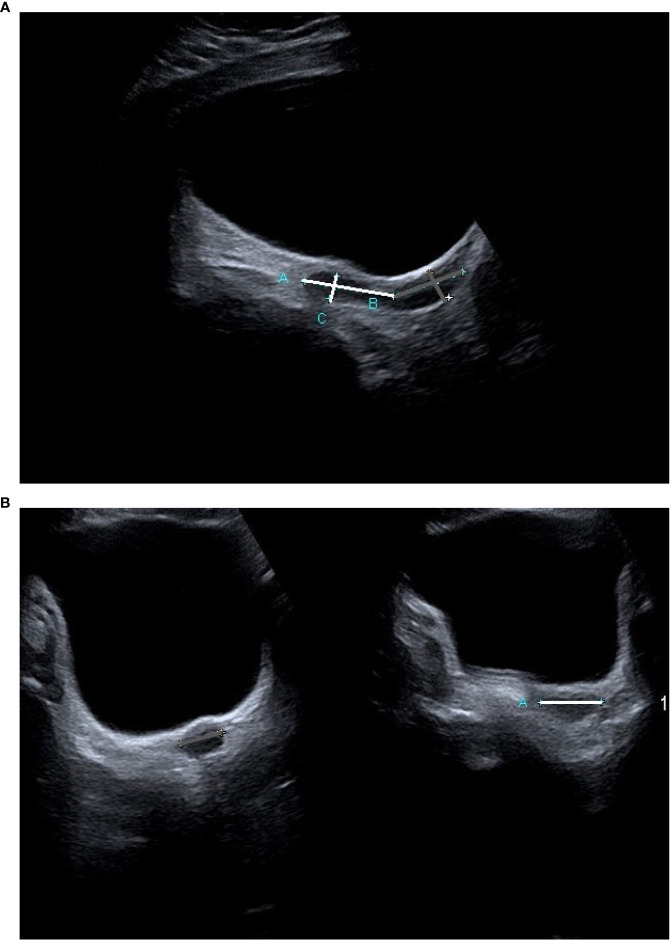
A transabdominal ultrasound image of the uterus in a TS patient prior to the beginning of ERT (A) longitudinal projection, (B) transverse projection). The measurements of the uterine body are marked white, and those of the cervix are marked grey.
